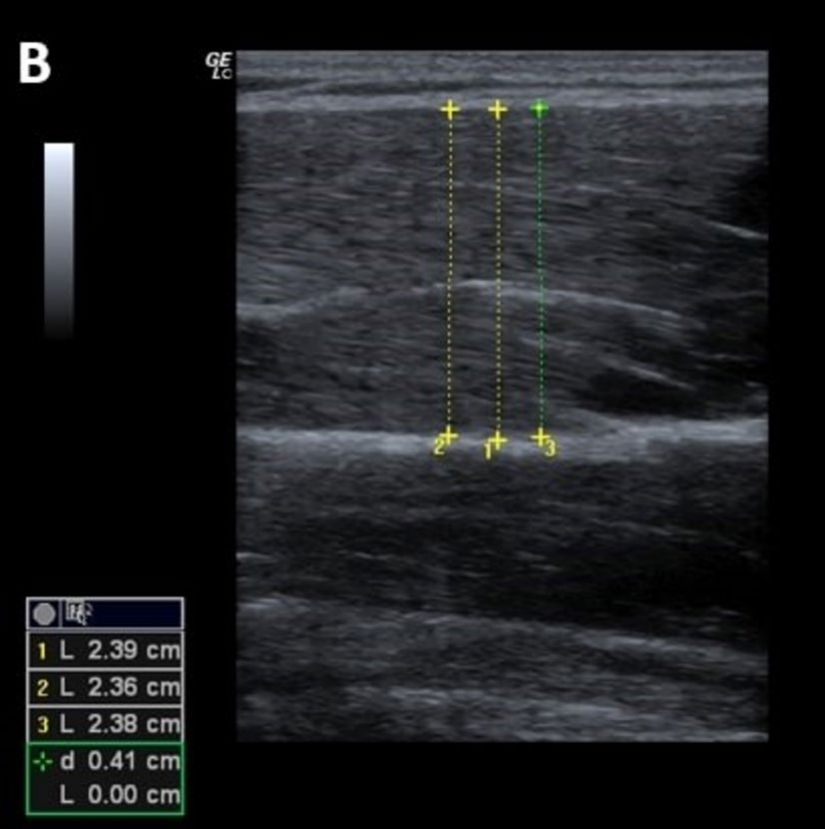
Sonography distance measurement of muscle thickness of pectoralis major muscle
